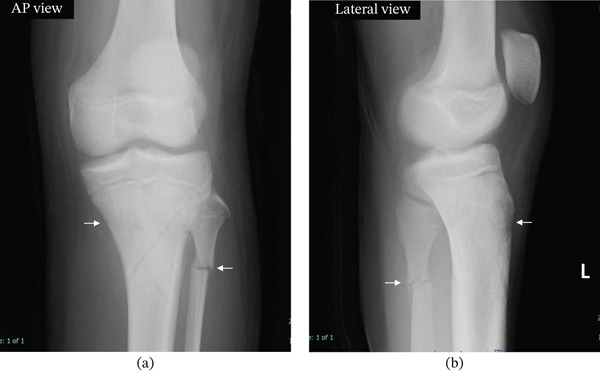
Plain x‐ray images of the left knee at 4 weeks postoperatively. (a, b) Radiographs of the left knee joint at 4 weeks postoperatively. The fracture lines are indicated by arrows. Both the left tibia and fibula show good callus formation with no displacement: (a) AP view and (b) lateral view.
